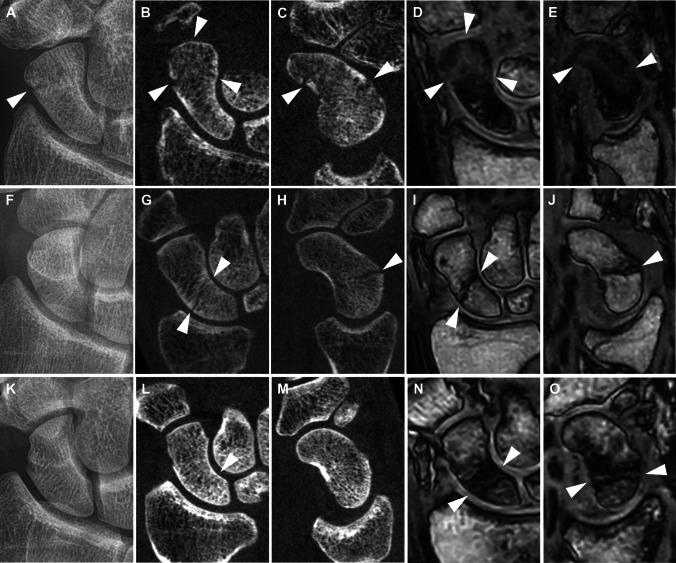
X-ray (A, F, K), CT (B/C, G/H, L/M), and high-resolution 3D T1-weighted MRI (D/E, I/J, N/O) of unstable scaphoid fractures. An extended oblique B1 type fracture in a 28-year-old male patient is shown in panels A-E, a slightly displaced transverse fracture type B2 in a 45-year-old male patient in F-J, and a fracture of the proximal third type B3 in a 37-year-old male patient in images K–O. Again, all fractures (indicated by arrowheads) are clearly detectable in the 3D T1-weighted sequence, in the first case accompanied by a marked edema (D/E). In contrast, the type B2 and B3 fractures are not visualized by X-ray (F/K) because radiographs were suboptimal due to pain. On CT, only a limited portion of the type B1 fracture is visible (G/H), whereas the type B3 fracture is barely discernible (L/M).In CT imaging the type B1 fracture is only partially visible (G/H), the type B3 fracture barely visible (L/M).
